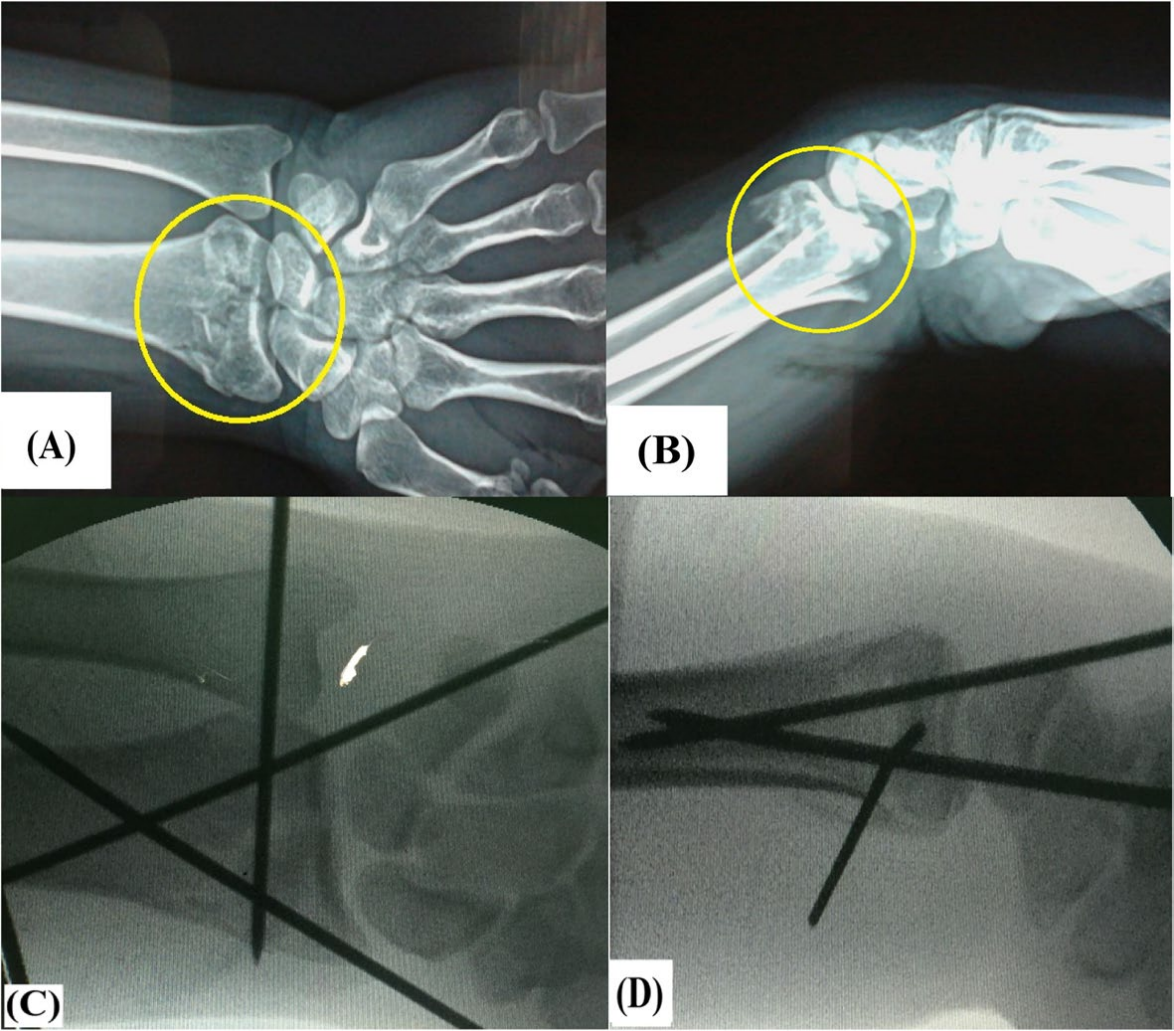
Demonstrate unstable intra-articular distal radius fracture in 56 years old female patient; A & B preoperative antero-posterior and lateral X-ray views of the fracture (marked in yellow circle); C & D intra-operative antero-posterior and lateral imaging intensifier views of triangular configuration of percutaneous K-wire fixation following closed reduction of the fracture
